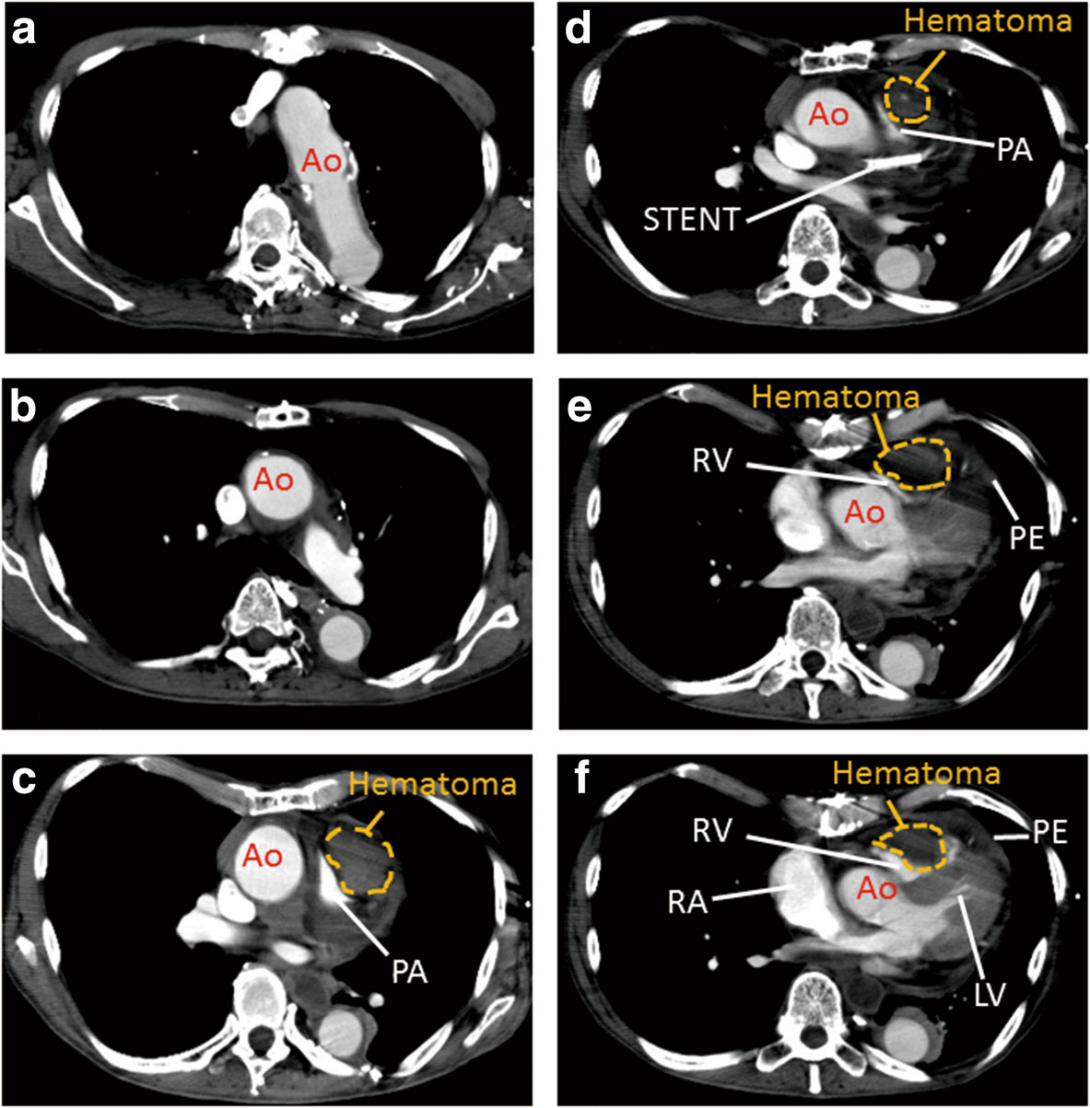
Contrast-enhanced computed tomography showing a massive epicardial hematoma without ascending aortic aneurysm, dissection, or rupture (a, b, c, d, e, f). The hematoma was compressing the pulmonary artery (c, d) and the right ventricle (e, f). Ao, aorta ; PA, pulmonary artery ; RA, right atrium ; RV, right ventricle ; LV, left ventricle; PE, pericardial effusion
